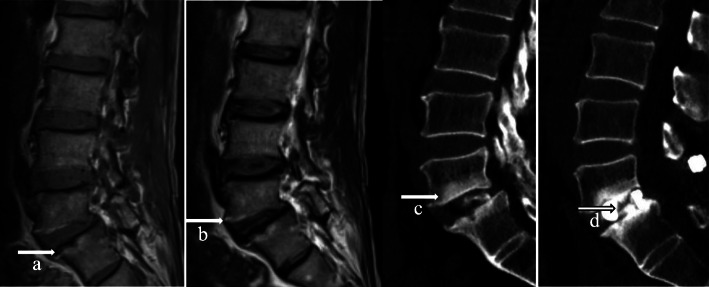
50–59-year-old patient with type 2 sclerotic Modic change (indicated by arrow)—high T1- and T2-weight magnetic resonance imaging (MRI) signals—showing the anatomical areas. a, b Sagittal T1-weighted (a) and T2-weighted (b) MRI scans show high signal at the L5-S1 endplates. Sagittal reconstructed (c) computed tomography (CT) scans show sclerosis at the L5-S1 endplates. Sagittal reconstructed (c, d) CT scans at the 12-month follow-up evaluationshow that there was no evidence of trabecular bone formation extending from the upper or lower endplate of L5-S1 (no fusion)
